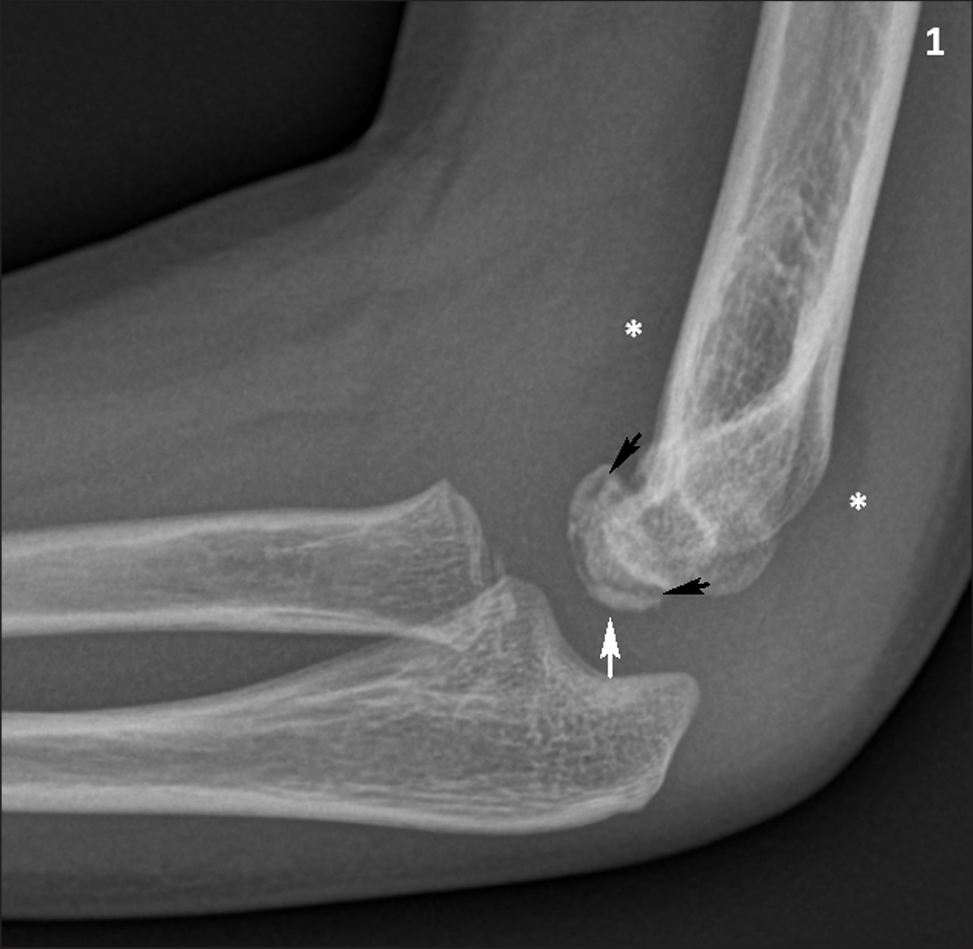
Conventional radiography of the right elbow, lateral view. The capitellum has a slightly irregular articular contour (white arrow) and there is a radiolucent line in the subchondral bone (black arrows). Note slight joint effusion with displacement of the elbow fad pads (asterisks).
